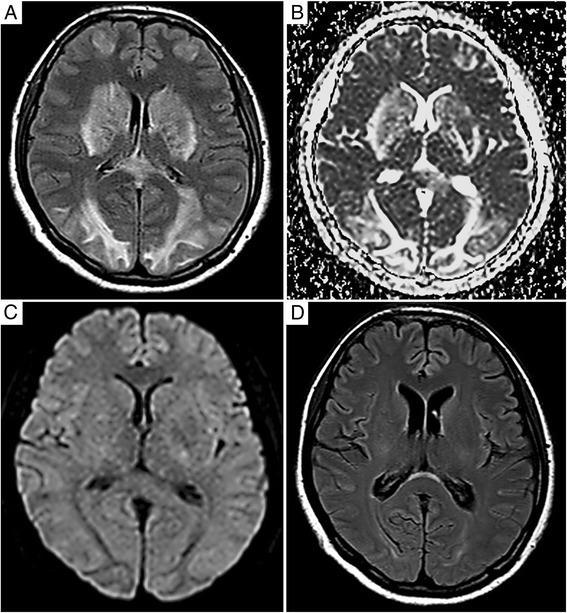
MRI showed hypersignal intense lesions in the cortical and subcortical white matter in the occipital lobes, basal ganglia and callosal splenium in both the fluid-attenuated inversion recovery (FLAIR) sequence (a) and apparent diffusion coefficient (ADC) (b), but these lesions were not recognized in diffusion-weighted imaging (DWI) (c). (b) The follow-up brain MRI, performed 2 weeks later, showed complete resolution of the lesions
